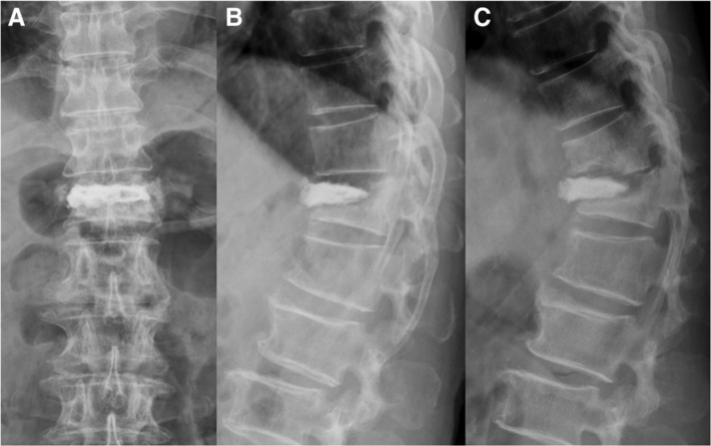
A 78-year-old man underwent PV for T12 osteoporotic VCF. The patient experienced intractable back pain and bilateral lower limb weakness after falling down and injuring himself. Anteroposterior radiograph (A) revealed a radiolucent line surrounding the bone cement. Lateral radiograph (B) revealed T12 bone cement anterior dislodgement. After 2 months of conservative treatment, the symptoms of back pain and leg weakness had worsened. The follow-up lateral radiograph (C) showed further cement dislodgement and T11 adjacent fracture with kyphotic deformity.
